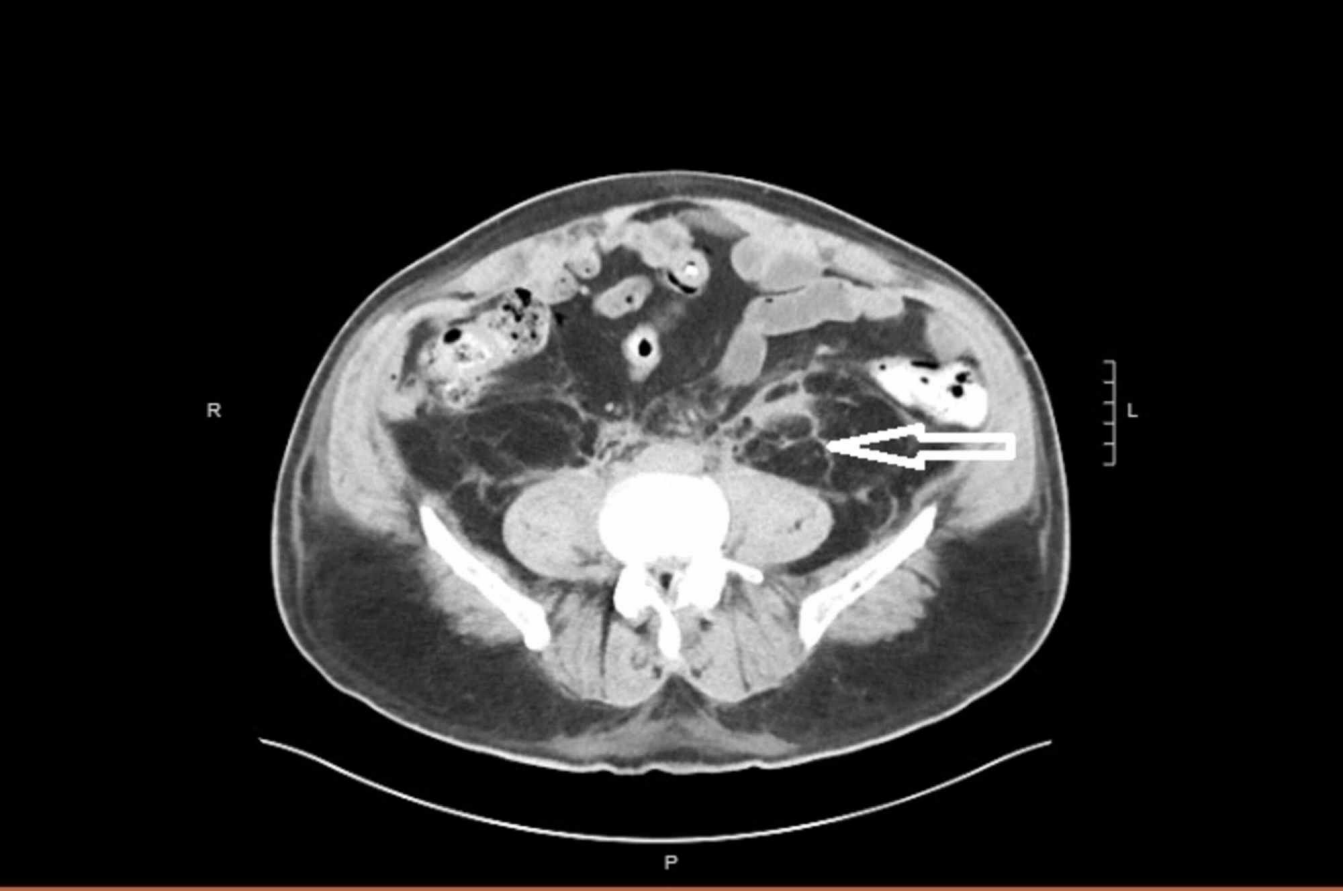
Cross-sectional CT shows the presence of retroperitoneal fibrosis with the depicted arrow.
